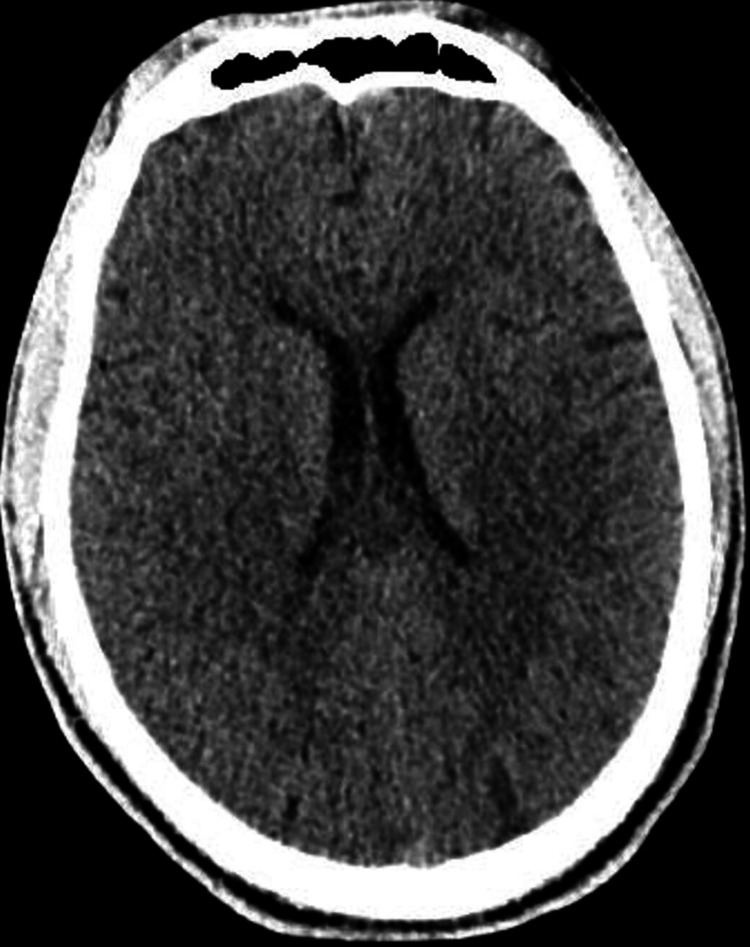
Head CT scan of the second patient (LB) This non-contrast axial head CT shows a normal study at the level of the frontal horns of the lateral ventricles. The frontal sinuses are well-aerated anteriorly, and the cerebral hemispheres appear symmetric with preserved gray-white matter differentiation, indicating no evidence of acute ischemia or cerebral edema. The lateral ventricles are normal in size and shape, with no signs of hydrocephalus or midline shift. The septum pellucidum is midline and intact. There is no evidence of intracranial hemorrhage, mass effect, or extra-axial collections. CT: computed tomography
